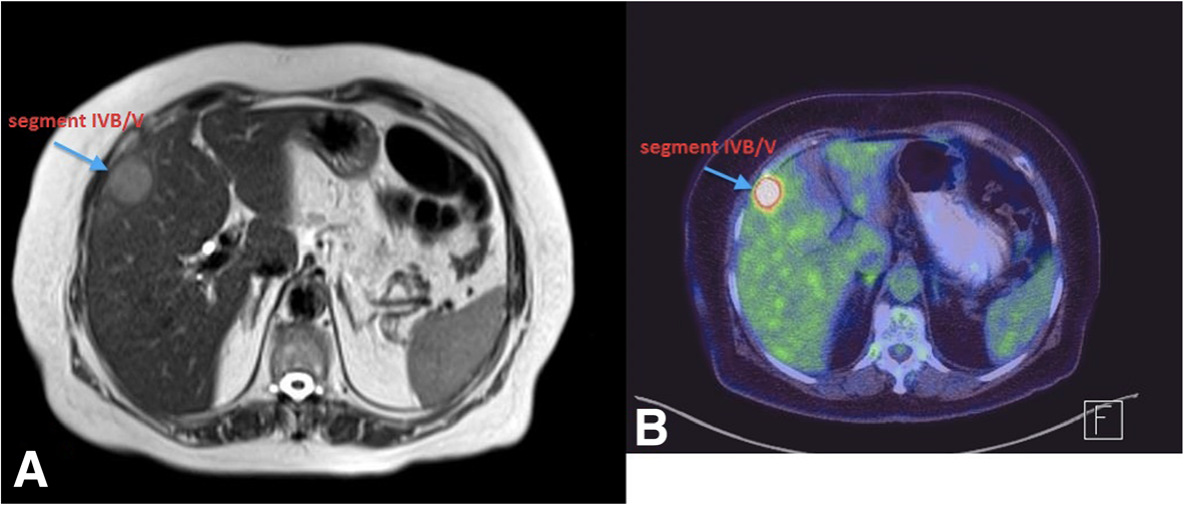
a MRI (prior to liver resection): a well-defined subcapsular hepatic focal lesion is noted at the right lobe segment IVB/V measures 28 × 27 × 30 mm, noted with capsule bulge with high T2 signal and low T1 signal. b PET CT (prior to liver resection): intensely hypermetabolic lesion in segment IVB/V of the liver consistent with malignancy just above the gallbladder fossa with slight contour bulge
